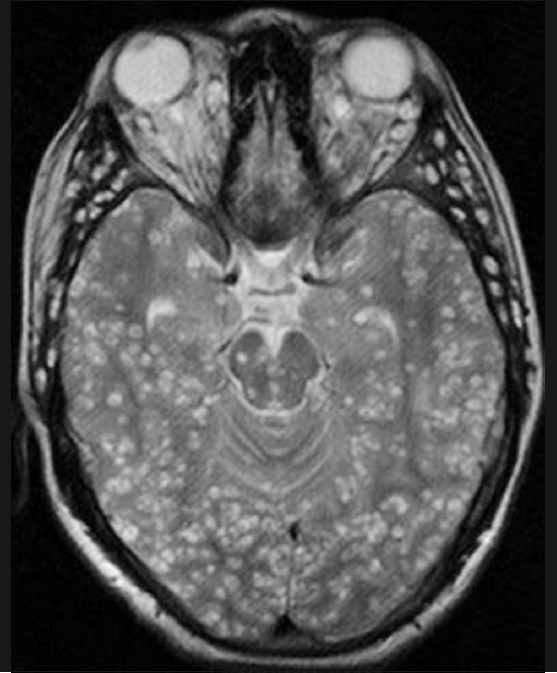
T2W axial MRI of the brain shows multiple cystic lesions with hypointense eccentric nodules, in both cerebral hemispheres, the midbrain, the cerebellum, and the extraocular muscles
